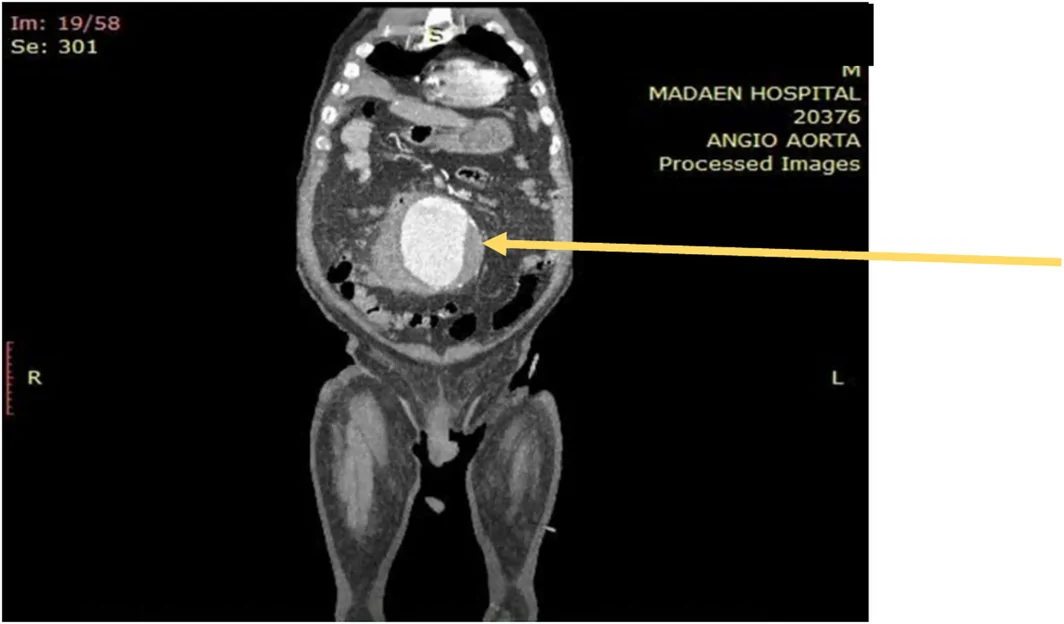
Coronal CT scan, ruptured and sealed‐off abdominal aortic aneurysm; shown by arrow.
